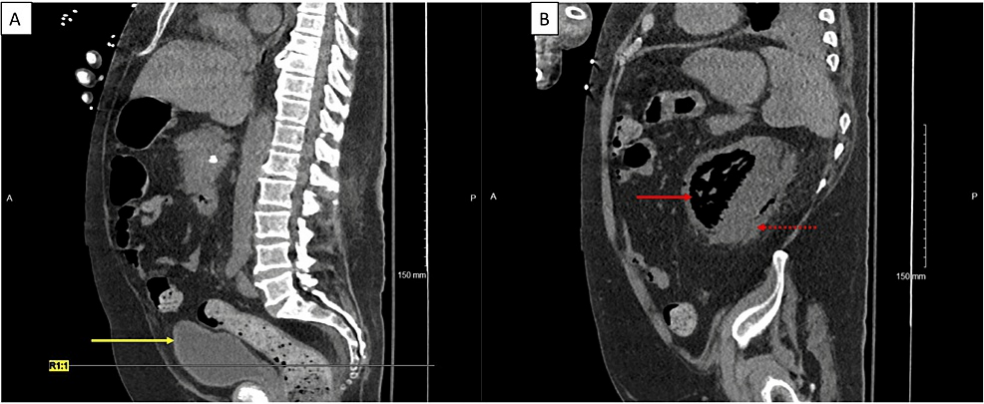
Non-contrast CT scan of abdomen and pelvis Panel A: Sagittal view showing fluid (urine) within the urinary bladder cavity and absence of gas within the wall of the urinary bladder indicating resolution of emphysematous cystitis (EC) (yellow arrow). Panel B: Sagittal view showing gas within the left renal parenchyma (solid red arrow), free fluid in the perinephric space (dotted red arrow), indicating persistence of emphysematous pyelonephritis (EPN).
